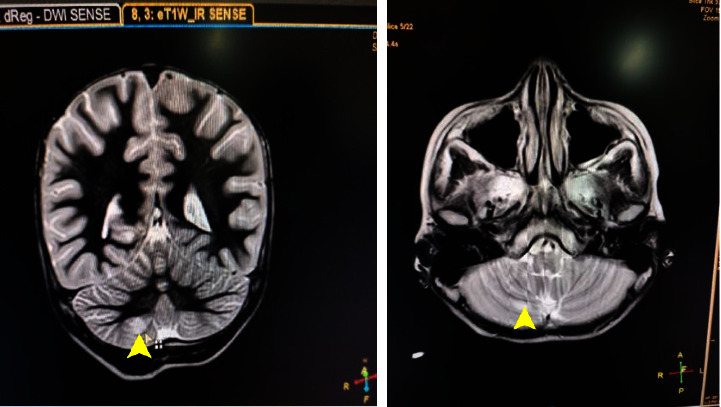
Subtle patchy areas (yellow arrow) of T2-FLAIR hyperintensities noted in inferomedial aspects of the right cerebellar hemisphere.
